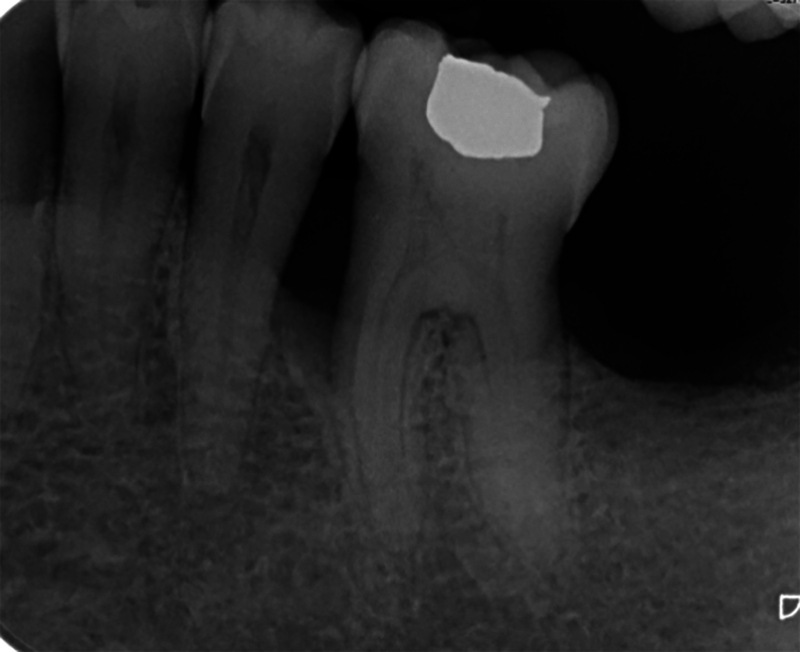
Clinical case no 2—Intraoral X-ray.
